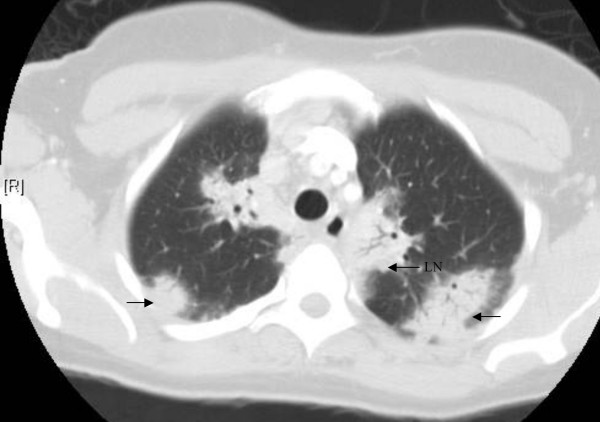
CT scan of the chest revealing peripheral consolidations and perihilar consolidations with hilar and mediastinal lymphadenopathy.
